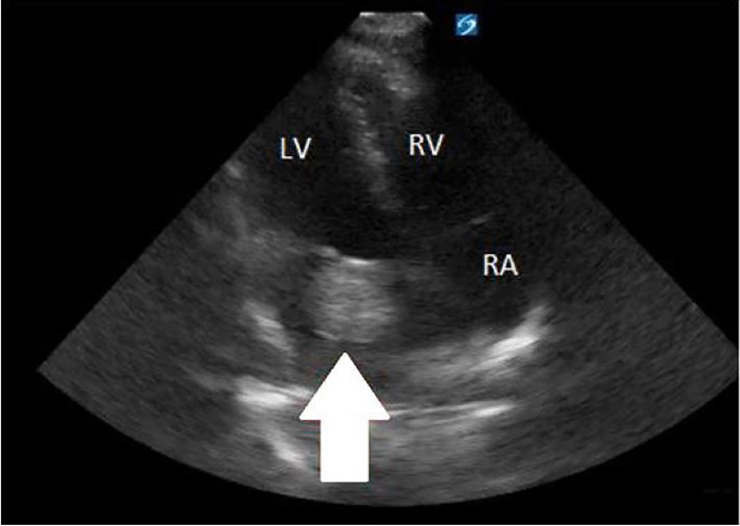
Apical four-chamber view of left atrial myxoma (arrow).
